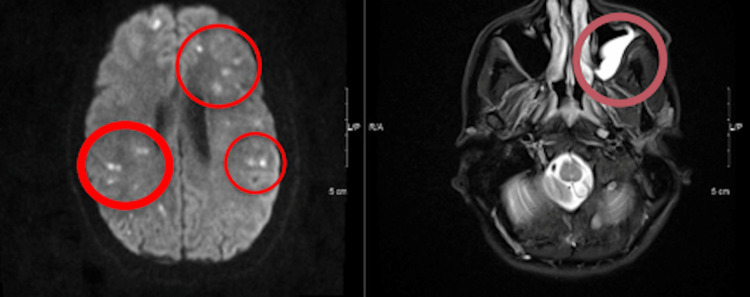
MRI (DWI sequence) demonstrates multiple foci of diffusion restriction (seen within red indicator regions on the left of the image) and (T2-sequence) left maxillary sinus thickening (within a single maroon indicator on the right of the image) DWI, diffusion-weighted imaging
